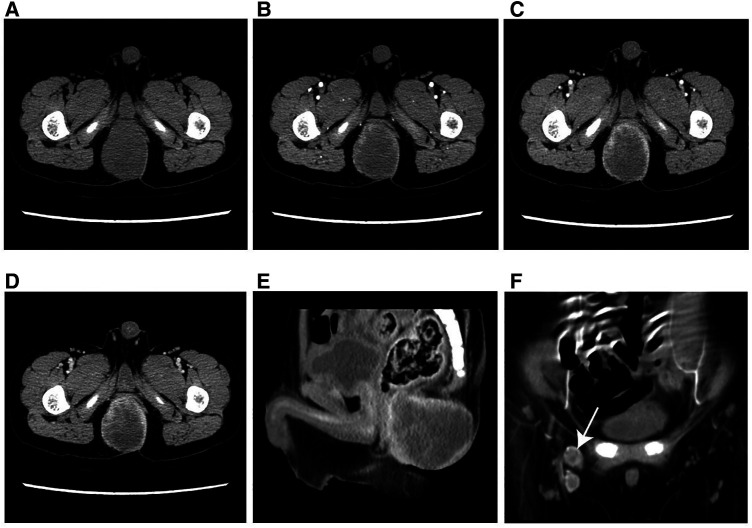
Typical example of RMS in a male infant aged 49 months (clinical manifestations: growing soft tisssue mass for 3 months). (A) The axial CT image revealed a well-defined, hypodense parenchymal mass measuring 47 mm in diameter. (B–D) On multi-phase enhancement, the CT values for each period were around 39 HU, 40 HU, and 60 HU, respectively, and displayed rim enhancement. (E) The sagittal CT image showed an indistinct boundary between the lesion and anterior rectum. (F) The coronal CT image presented enlarged lymph nodes in the right groin area (arrow).
